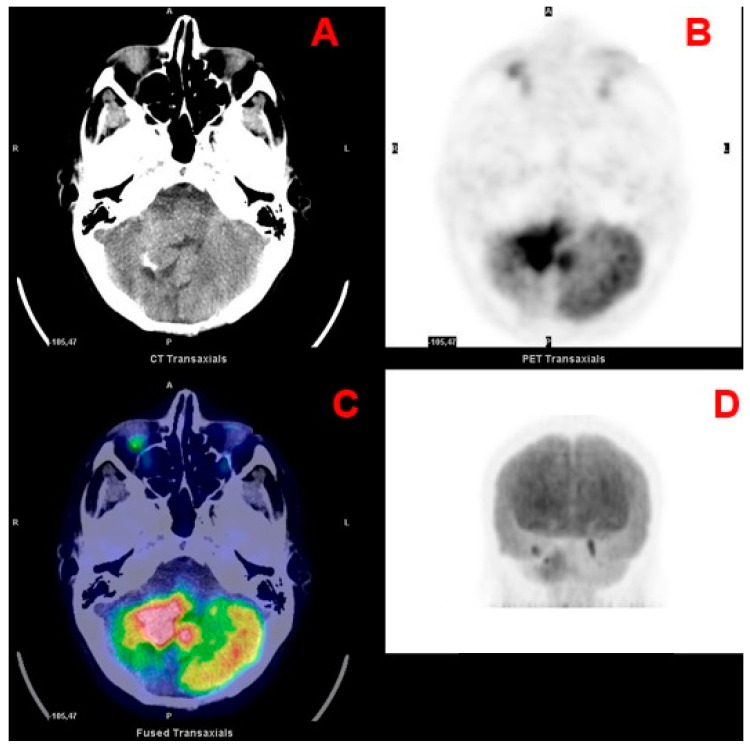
Transaxial CT (A), PET (B) and fused PET/CT (C) and maximum intensity projection (D) images of a medulloblastoma.
